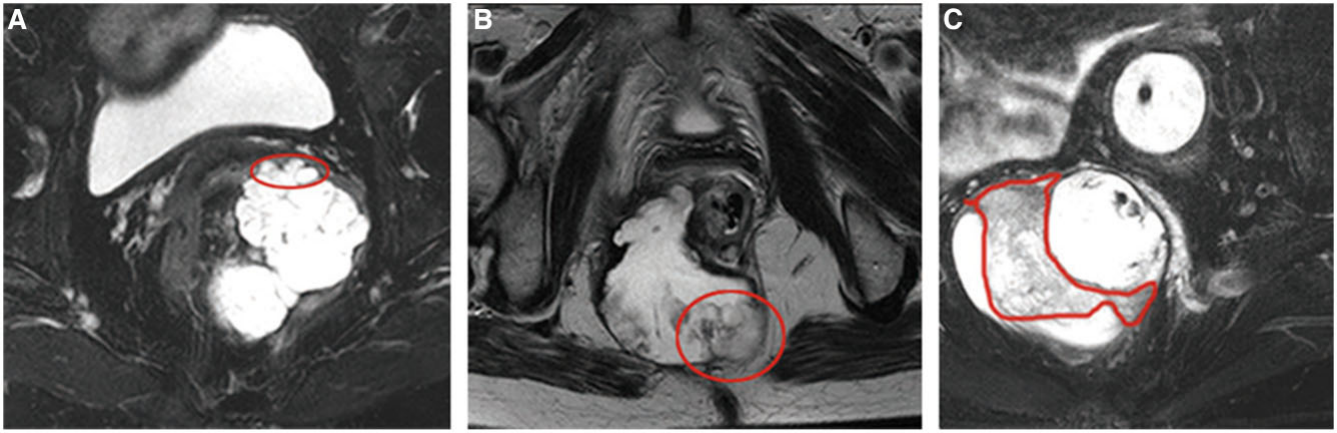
Magnetic resonance imaging (MRI) features of the solid portions of malignant tumours. (A) Mucin forms a major component of primary mucinous adenocarcinoma. The tumour has no capsule, showing a high signal intensity on fat-suppressed T2-weighted MR images. (B) The wall of the epidermoid cyst with components of a squamous-cell carcinoma is damaged and irregular, and the lobulated contour has surface projections, which exhibit slightly higher signal intensity on T2-weighted images. (C) There are intermediate-intensity portions of mucinous adenocarcinoma arising from the tailgut cyst on fat-suppressed T2-weighted MR images. The malignant components are circled in red.
